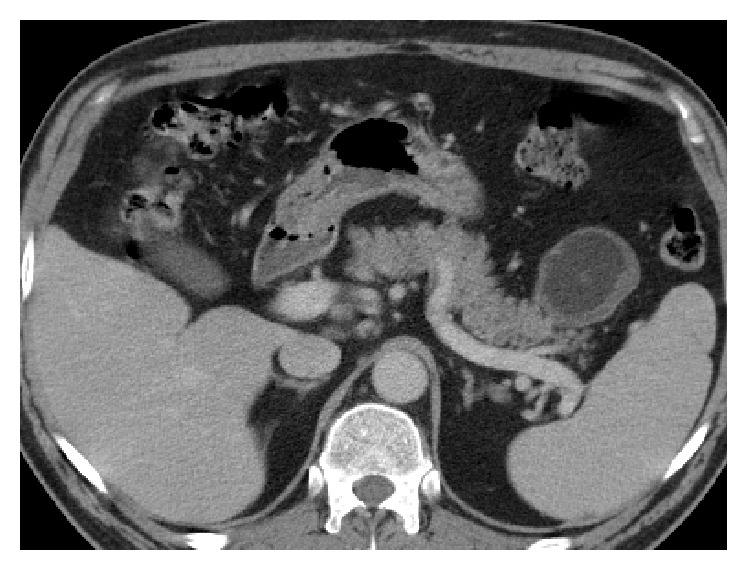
Computed tomography (CT) of the abdomen showing a complex solid cystic mass in the distal pancreatic tail.
